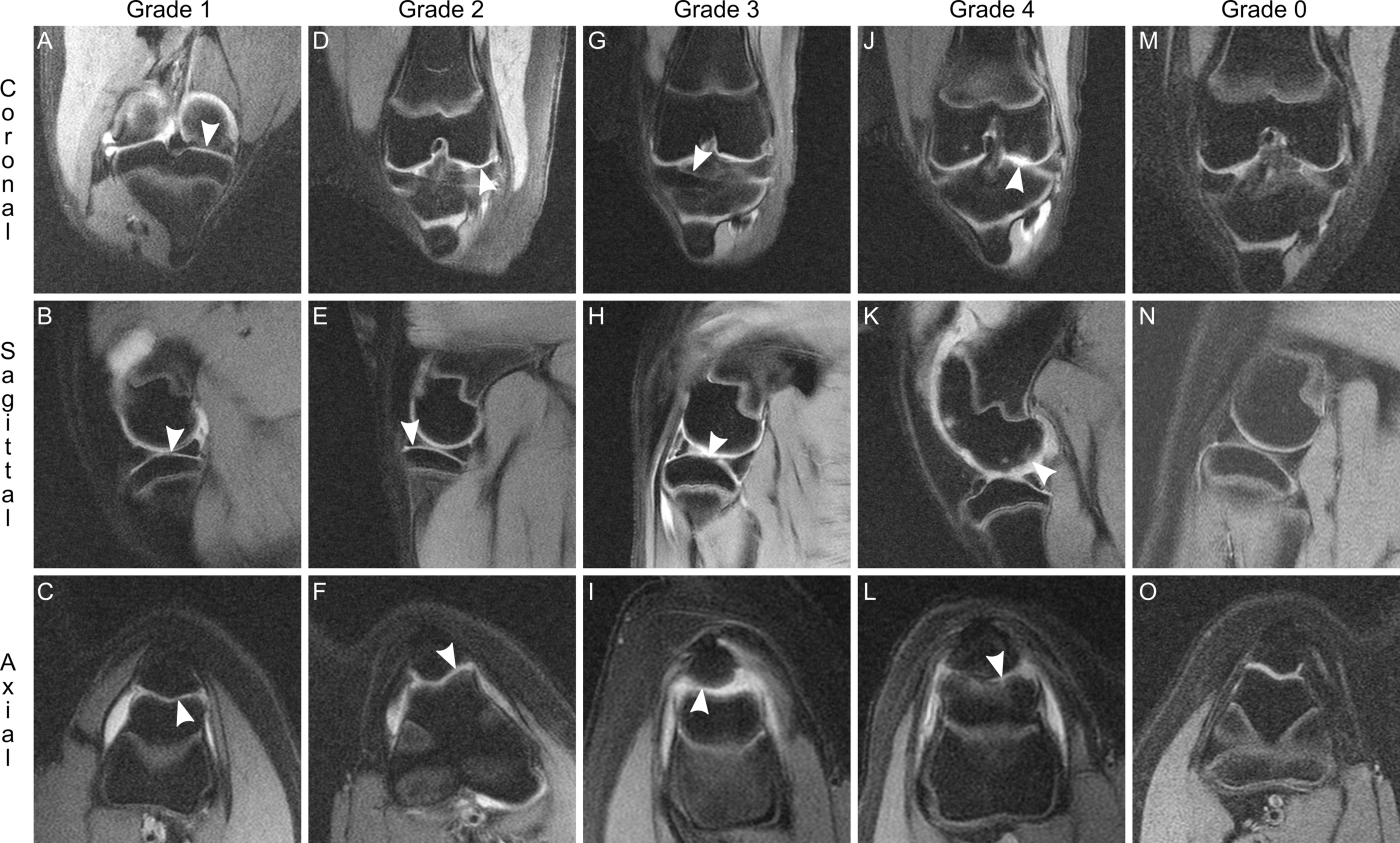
Graded destruction of articular cartilage. Articular cartilage was seen on multiplanar MR images as a band hyperintense (white) signal between the intraarticular space and subchondral bone. The thickness of this band was compared throughout its length and the quality and degree of its thinning was used to grade each cartilage lesion. Grade 1 damage overlying the tibial plateau (A and B) and patella (C), as indicated by nearly normal appearance with superficial indentation (arrowheads), here from one 4 mg knee at week 8. Grade 2 damage overlying the femoral condyle (D), tibial plateau (E), and trochlea (F), as indicated by cartilage thinning of less than 50% (arrowheads), here from two 4 mg knees at weeks 8 and 9. Grade 3 damage overlying the tibial plateau (G and H) and patella (I), as indicated by loss of cartilage of at least 50% and up to subchondral bone but not deeper (arrowheads), here from two 4 mg knees at weeks 6 and 8. Grade 4 damage overlying the femoral condyle (J and K) and trochlea (L), as indicated by complete loss of cartilage with associated underlying damage to subchondral bone (arrowheads), here from one 4 mg knee at week 12. Representative Grade 0 images from one non-injected knee contralateral to a 4 mg knee at week 12 (M, N, O) demonstrate full thickness cartilage throughout all articular surfaces. Images are representative of n = 587 cartilage lesions identified. Coronal views: top row. Sagittal views: middle row. Axial views: bottom row.
